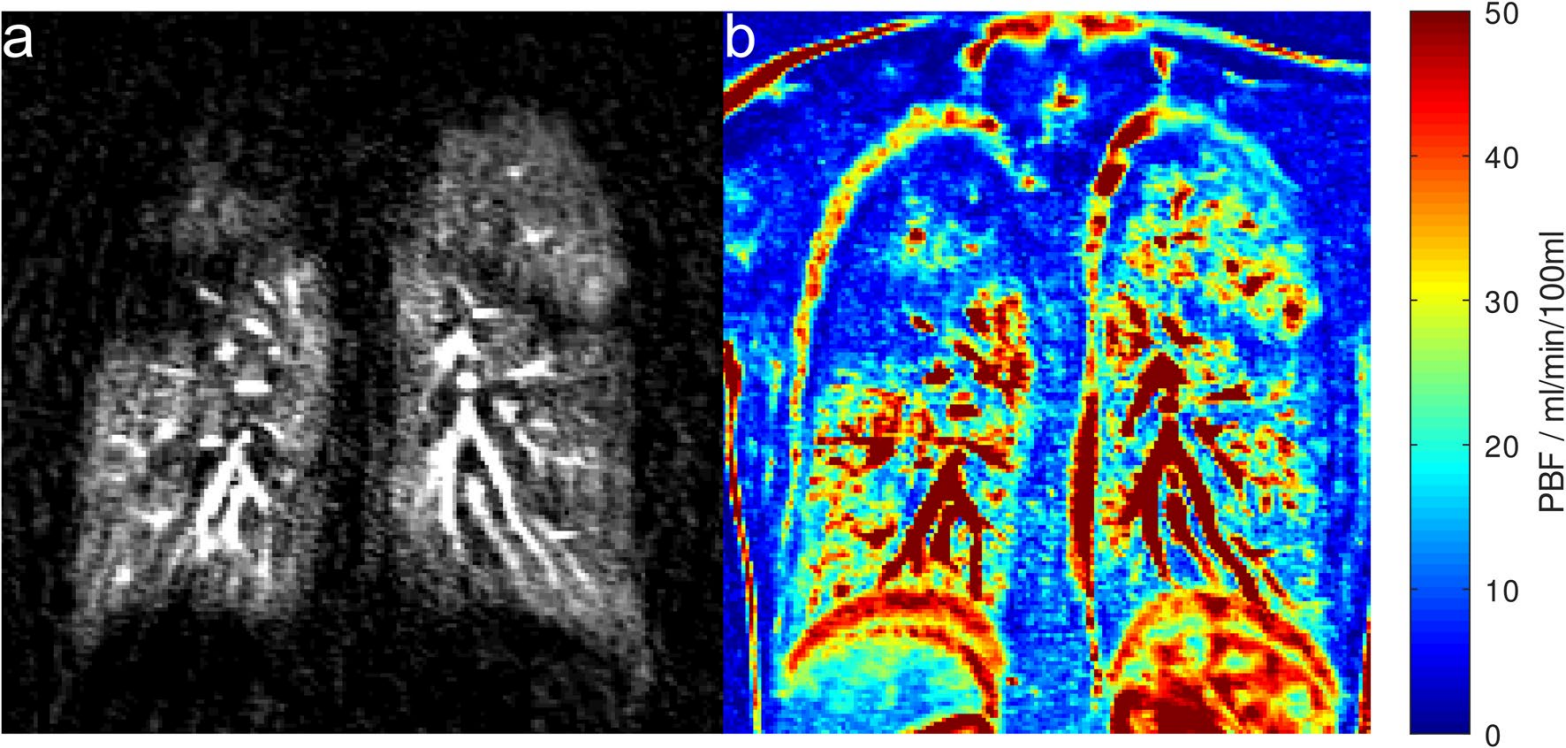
(a) Maximum enhancement in a series of first‐pass dynamic contrast‐enhanced MR images as well as pulmonary blood flow map (b) in a pediatric cystic fibrosis patient (17‐year‐old female, FEV_1_ 100% of predicted value). Perfusion defects are readily apparent mainly in the right lung. FEV_1_, forced expiratory volume in 1 s; PBF, pulmonary blood flow.
